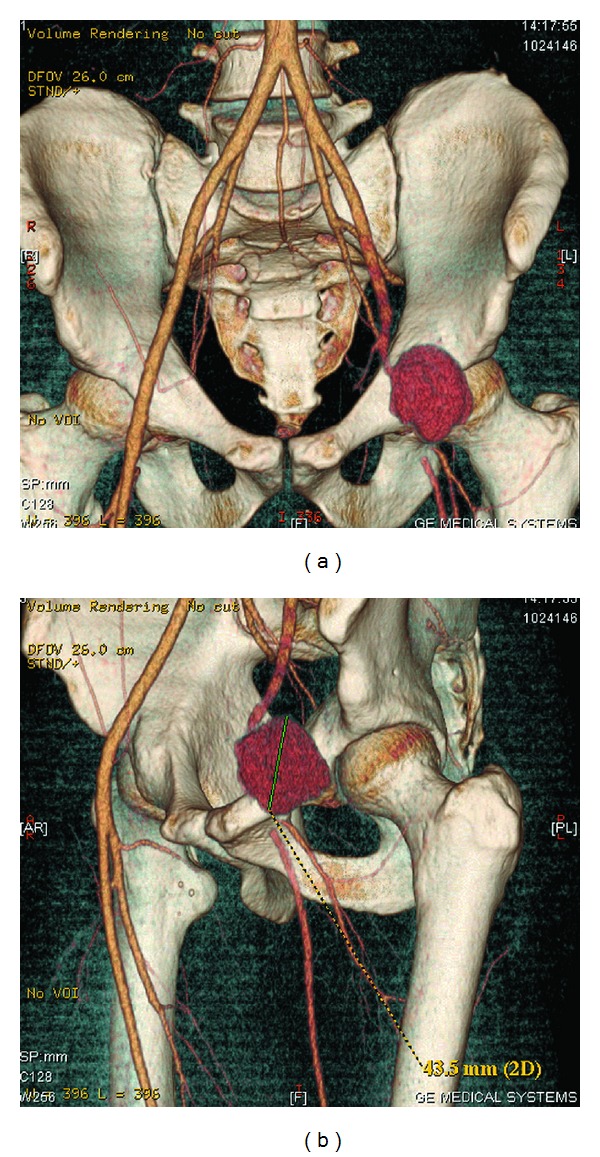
CT angiography: pseudoaneurysm of the external iliac artery with acute arterial thrombosis in the common femoral artery bifurcation.
